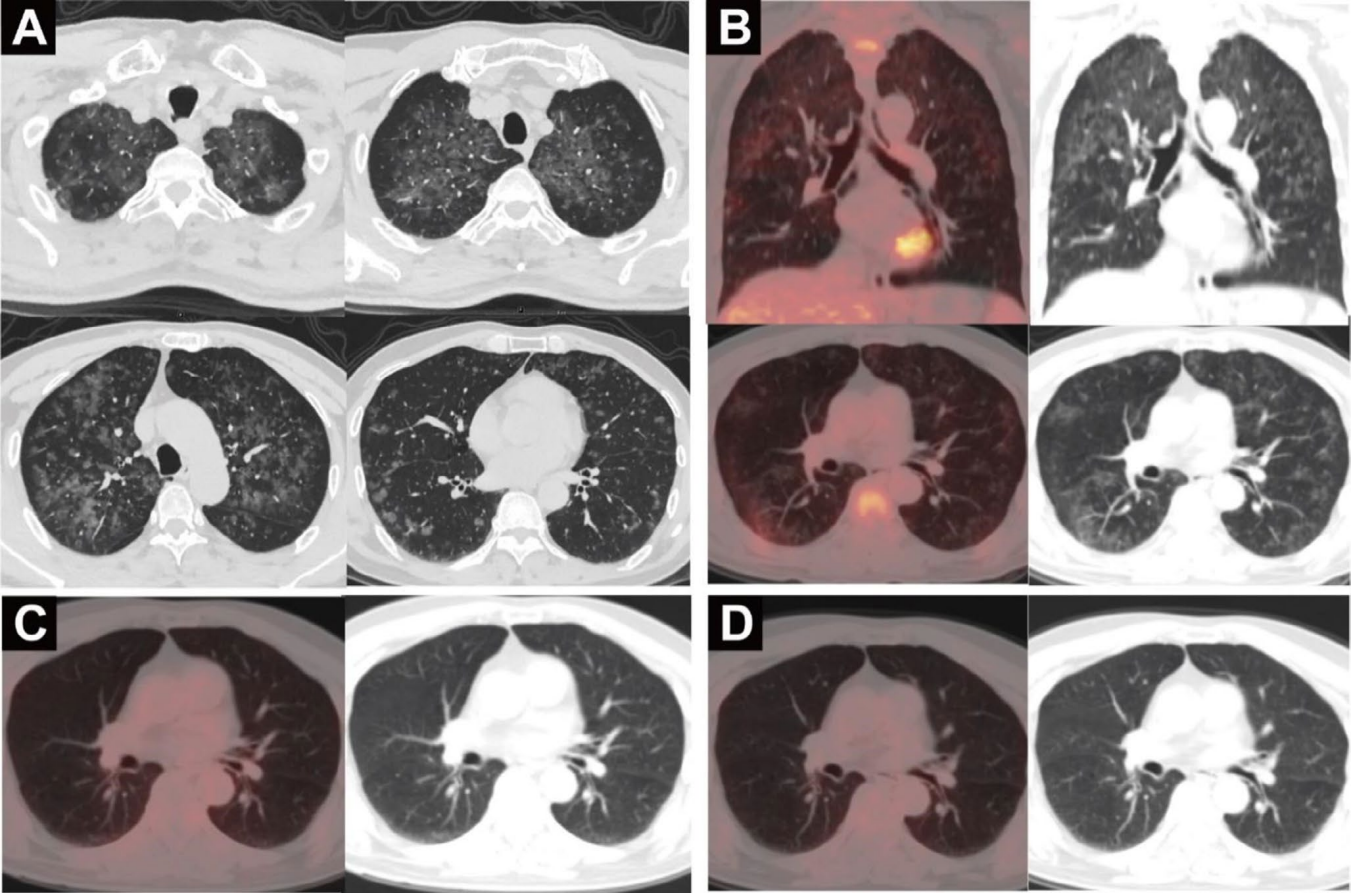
(A) Chest CT images before chemotherapy showing bilateral diffuse patchy and nodular GGOs of the lungs; (B) FDG PET/CT images before chemotherapy showing increased FDG uptake; FDG PET/CT after four (C) and eight (D) rounds of chemotherapy showing that previous lesions disappeared and no FDG uptake was observed.
